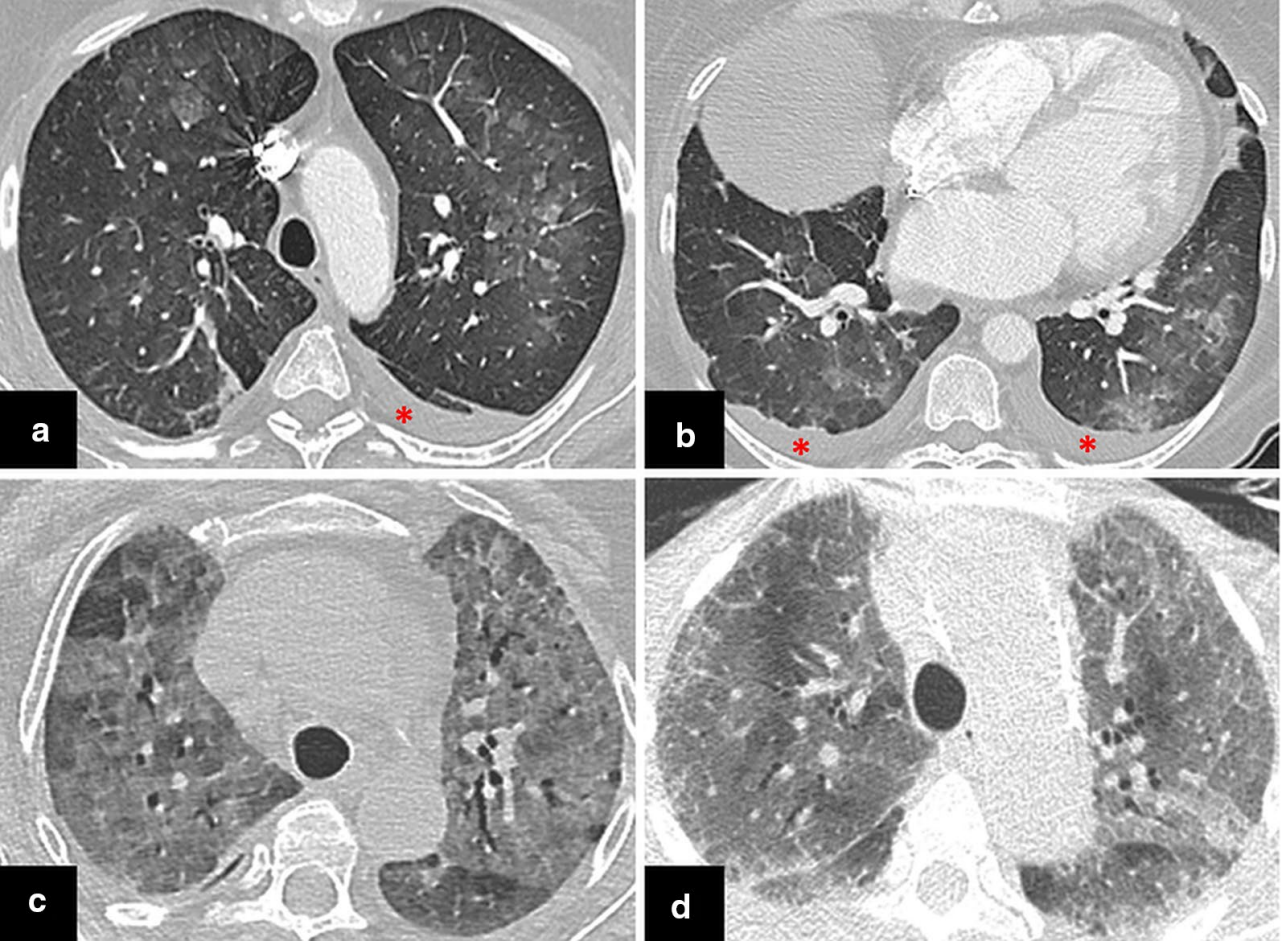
Distribution of ground-glass opacities in pulmonary edema and COVID-19 pneumonia. a, b Bilateral predominantly central GGOs are observed in a patient with pulmonary edema. Subpleural sparing is seen. There is also cardiomegaly and bilateral pleural effusion (asterisks). c, d In two different COVID-19 patients, diffuse GGOs are seen in bilateral upper lobes on axial CT images. The absence of ancillary findings such as pleural effusion and cardiomegaly may help in the differential diagnosis
